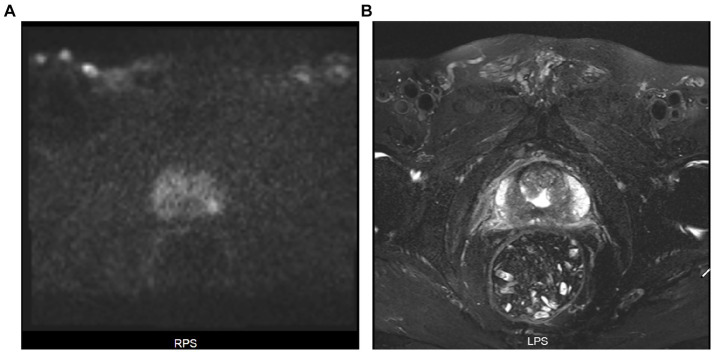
(A) Axial diffusion-weighted imaging (DWI) sequence of the prostate (phase encoding direction: RPS), where the hyperintense signal area indicates the prostate cancer lesion; (B) Axial T2-weighted enhanced magnetic resonance imaging (MRI) of the prostate (phase encoding direction: LPS), where the irregularly enhanced central area is the primary focus of prostate cancer.
